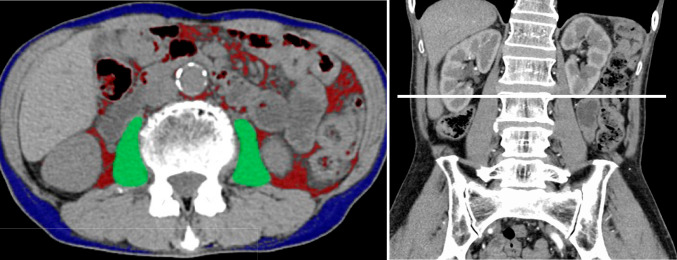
Skeletal muscle was evaluated by the areas of psoas muscle at the third lumbar vertebra level on CT image, and the area of PM was automatically drawn using Synapse Vincent (green area)
